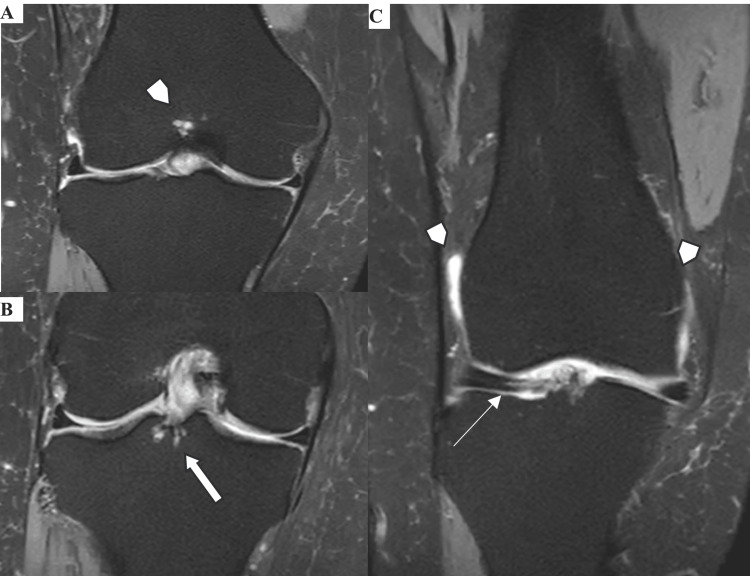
Associated MRI findings of mucoid degeneration of the anterior cruciate ligament Coronal PD-FS images (A) Intraosseous cyst at the femoral ACL footprint (arrowhead). (B) Intraosseous cyst at the tibial ACL footprint (arrow). (C) Stoller grade III signal in the anterior horn of the lateral meniscus consistent with a meniscal tear (arrow), with small joint effusion (arrowheads). PD-FS: proton density fat-saturated
